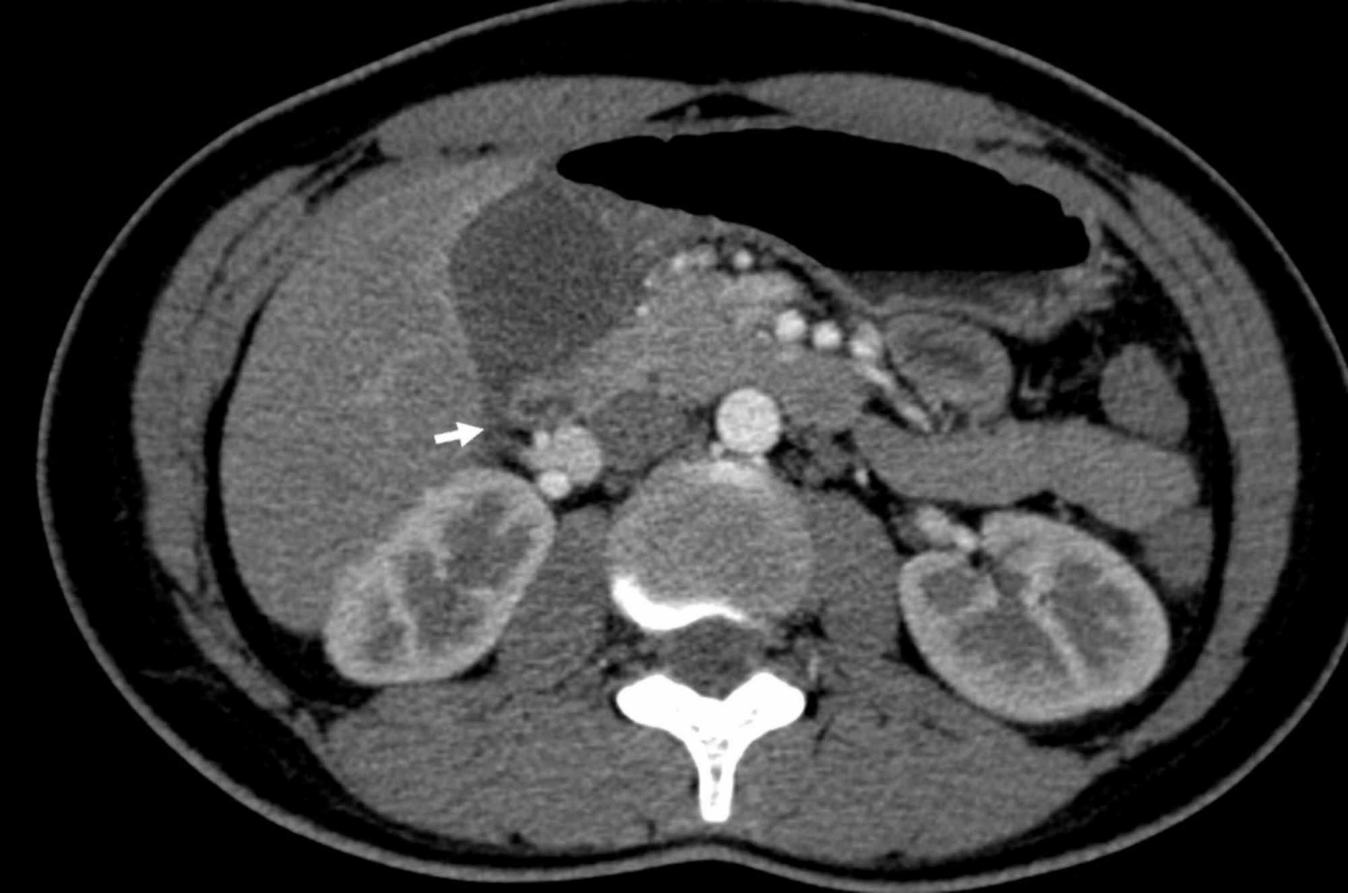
Abdominal enhanced CT The enhanced CT demonstrated a distended gallbladder measuring 82 x 42 mm, and a non-enhanced wall associated with a pericholecystic effusion (arrow). Abbreviation: CT, computed tomography
